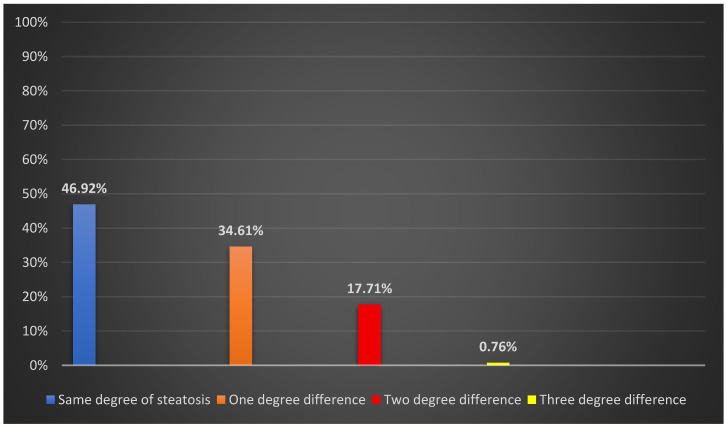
Distribution of concordant and discordant steatosis grading between ultrasound and CAP.
